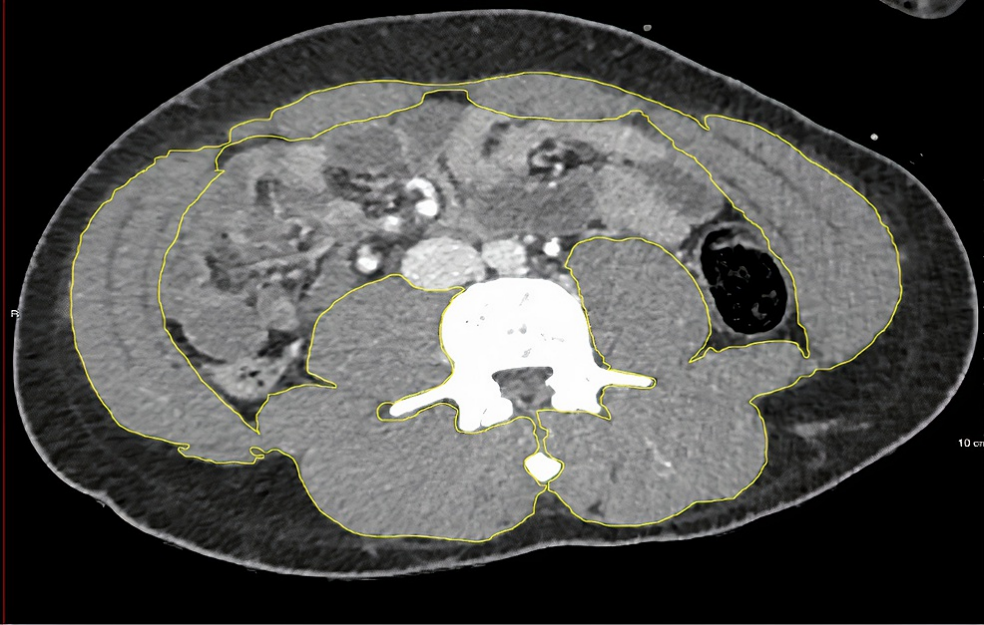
Axial CT image of total skeletal muscle area delineated in yellow at the level of the L3 vertebra
